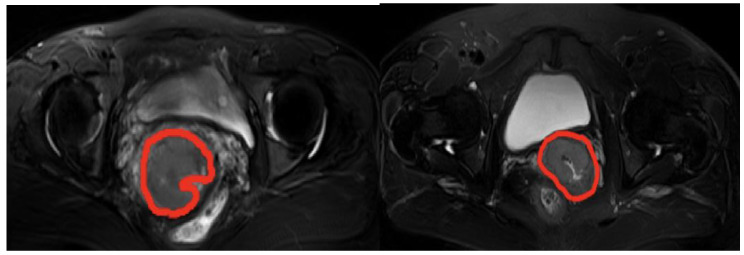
MRI images of a patient with pCR before radiotherapy (left) and a patient with non-pCR before radiotherapy (right). We used T2WI-FS here to provide a clearer image of the collected data; The red circles represent the tumor area.
